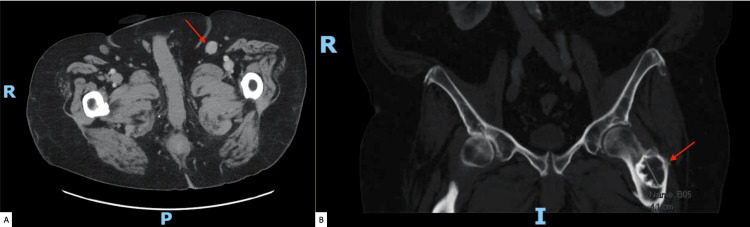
CT scan of the abdomen and pelvis reveals a prominent left groin lymph node measuring 1.8 cm (a) and a 4.1 cm lesion in the proximal left femur (b), both highlighted by a red arrow.
